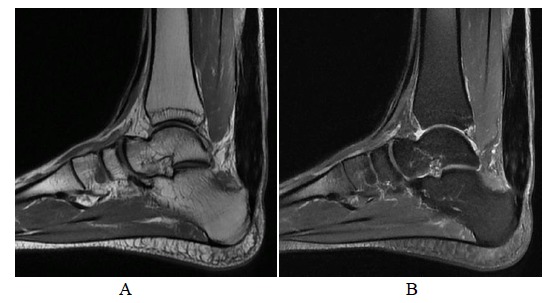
6 months after open surgery for Achilles repair in Patient A. The outline of repaired tendon is now well defined with hypointense scar tissue across the surgical site on PD-weighted and fat suppressed T2-weighted sagittal images.
